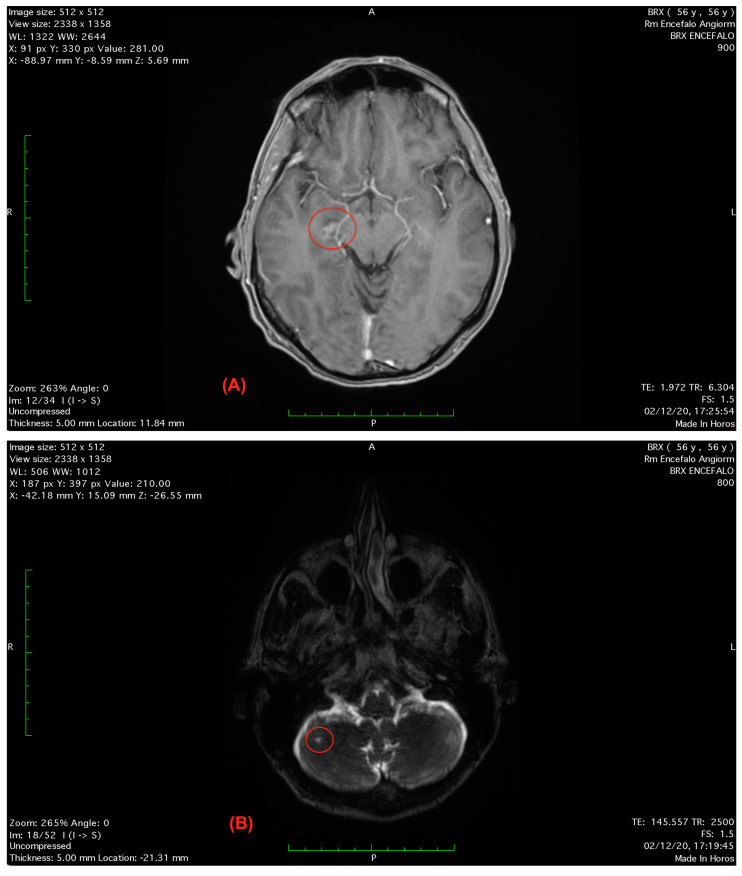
(A) Axial T1-weighted FLAIR scan showing a small hyper-intense areola in correspondence to the lower right midbrain’s colliculus. (B) Axial T2-weighted brain magnetic resonance imaging scan showing the small hyper-intense areola in the right cerebellar hemisphere.
